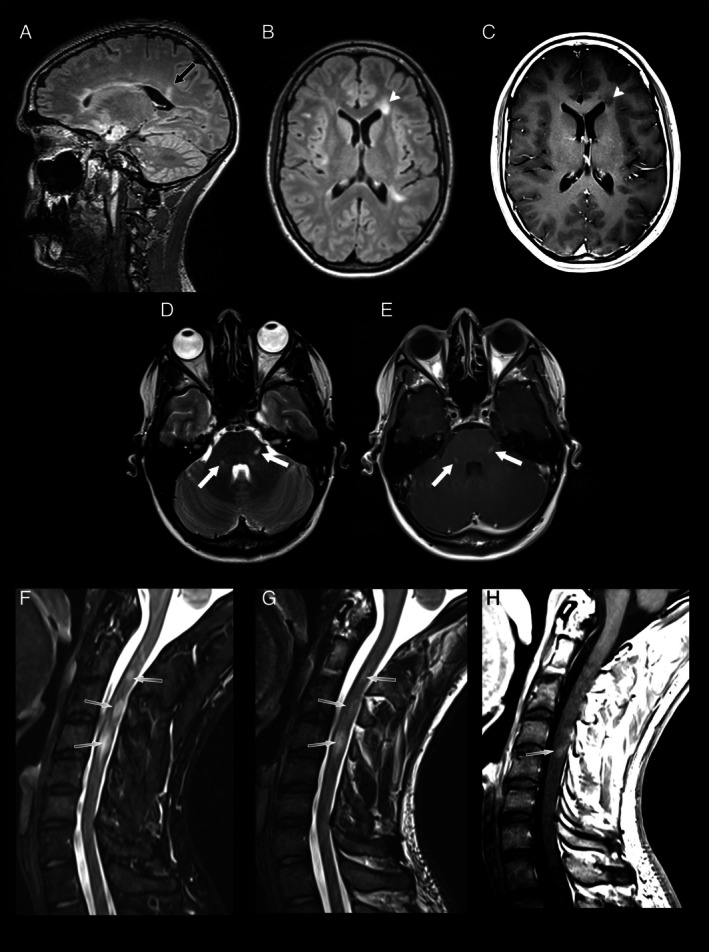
The image acquisition protocol followed the MAGNIMS‐CMSC‐NAIMS recommendations and included a (A) 3D FLAIR sequence with (B) multiplanar reconstruction, an axial T1 TSE (C and E) after contrast media injection, an (D) axial T2 TSE for brain imaging as well as a (F) sagittal T2 TSE STIR, (G) T2 TSE, and (H) T1 TSE after contrast media injection in separate acquisitions for the cervical as well as the thoracic (not shown) spine. In this 24‐year‐old female patient, periventricular lesions (black arrow) with partially lowered T1 signal (arrow heads) as well as infratentorial lesions showing contrast enhancement (white arrows) as well as lesions in the cervical spinal cord (gray arrows), in part with contrast enhancement were observed. Discrepancies between different readers were mainly due to different interpretations of lesions as being in the deep white matter or in juxtacortical or periventricular location.
